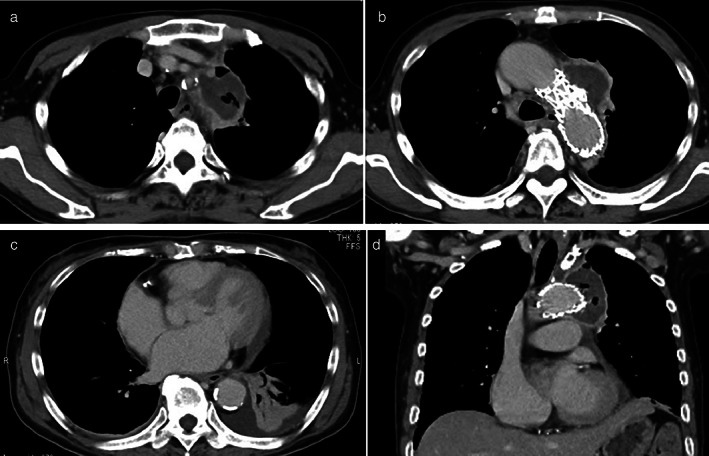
Contrast‐enhanced CT after thoracic endovascular aortic repair (TEVAR). (a–c) Axial images. (d) Sagittal images. No stent migration or endoleak is observed, and the aneurysm is reduced.
